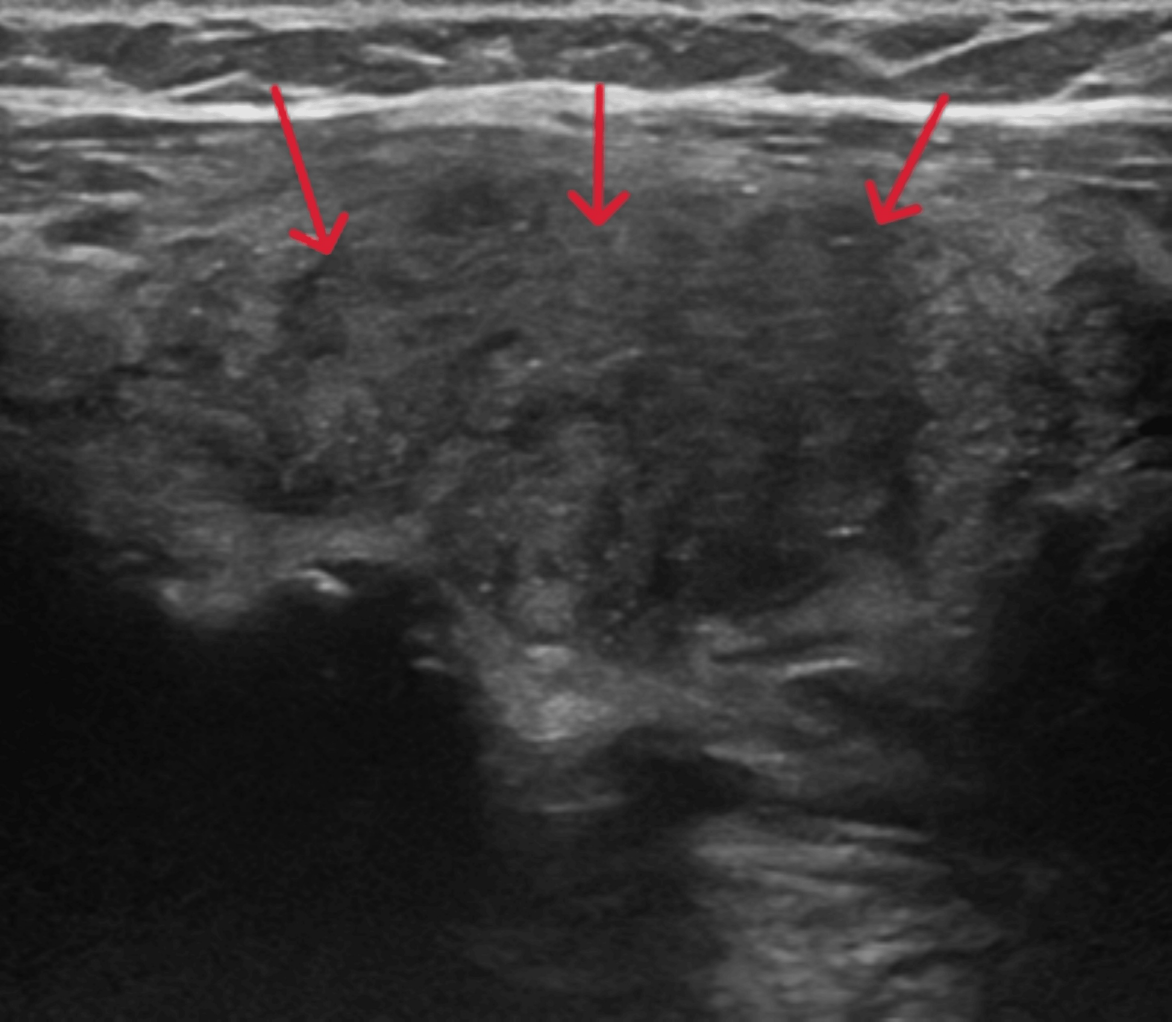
Ultrasound scan of the left lobe of thyroid depicting a bulky thyroid (red arrows) with diffuse disease
